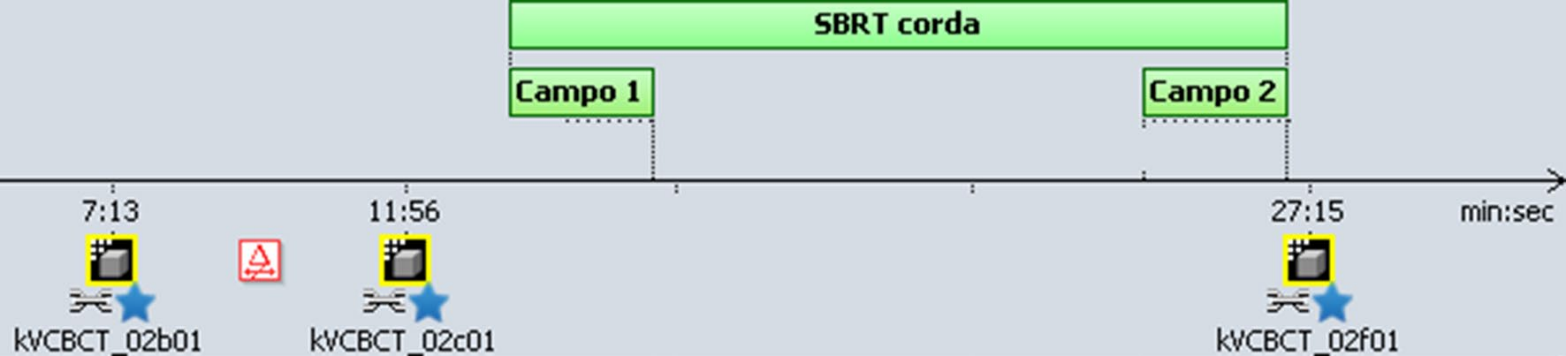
The sequence of image acquisition: a CBCTsetup is acquired, eventually setup corrections are applied, CBCTpre is acquired, at the completion of the irradiation CBCTpost is acquired
